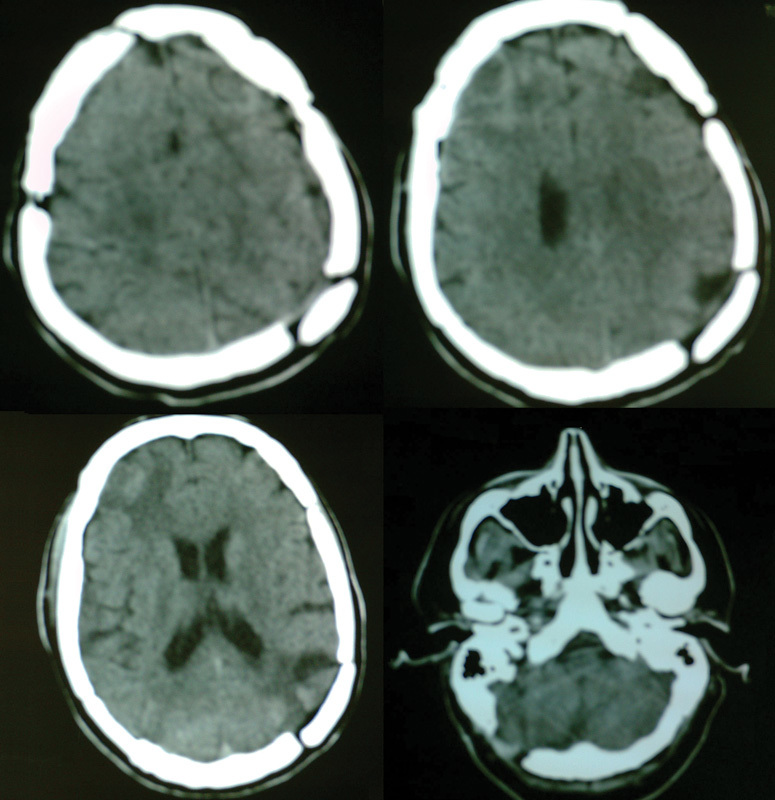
Postoperative cerebral computed tomography scans.
